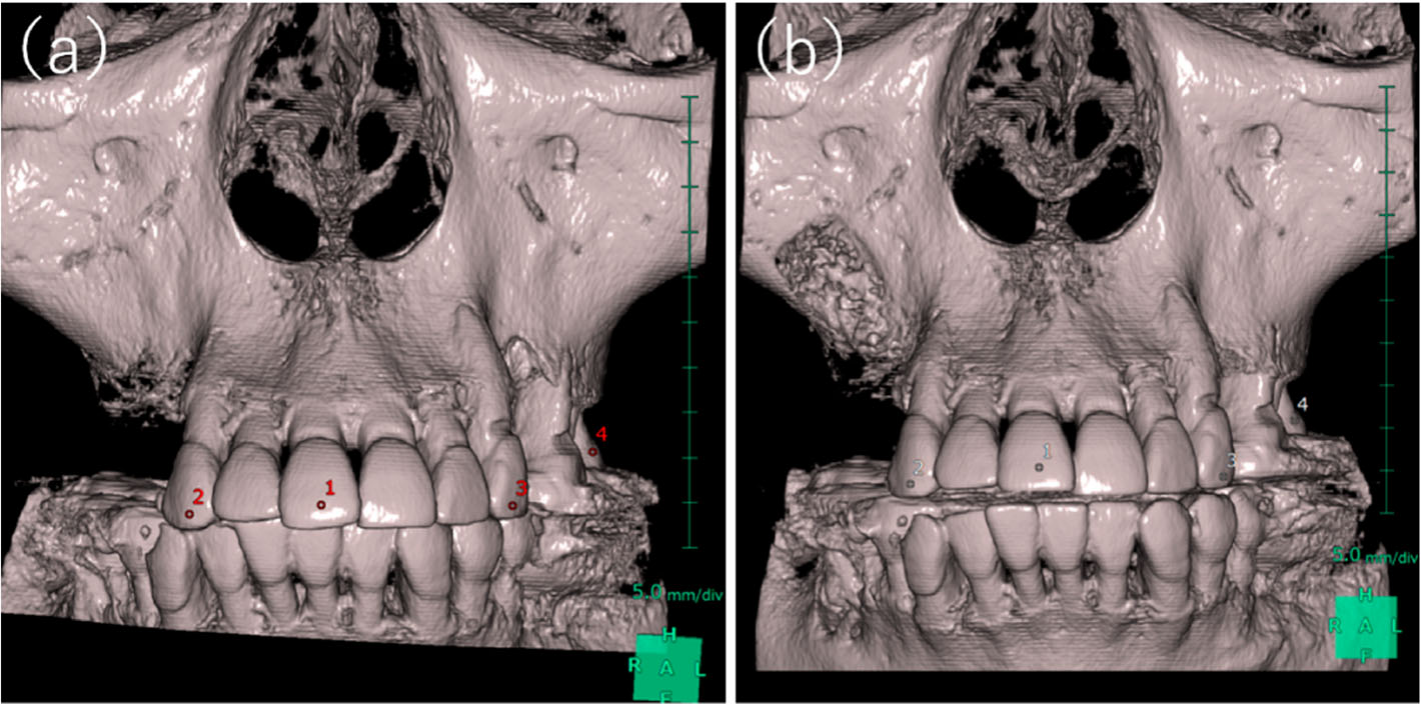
“Manual alignment” was performed using residual teeth as reference points. a Preoperative CBCT data. b Postoperative CBCT data
